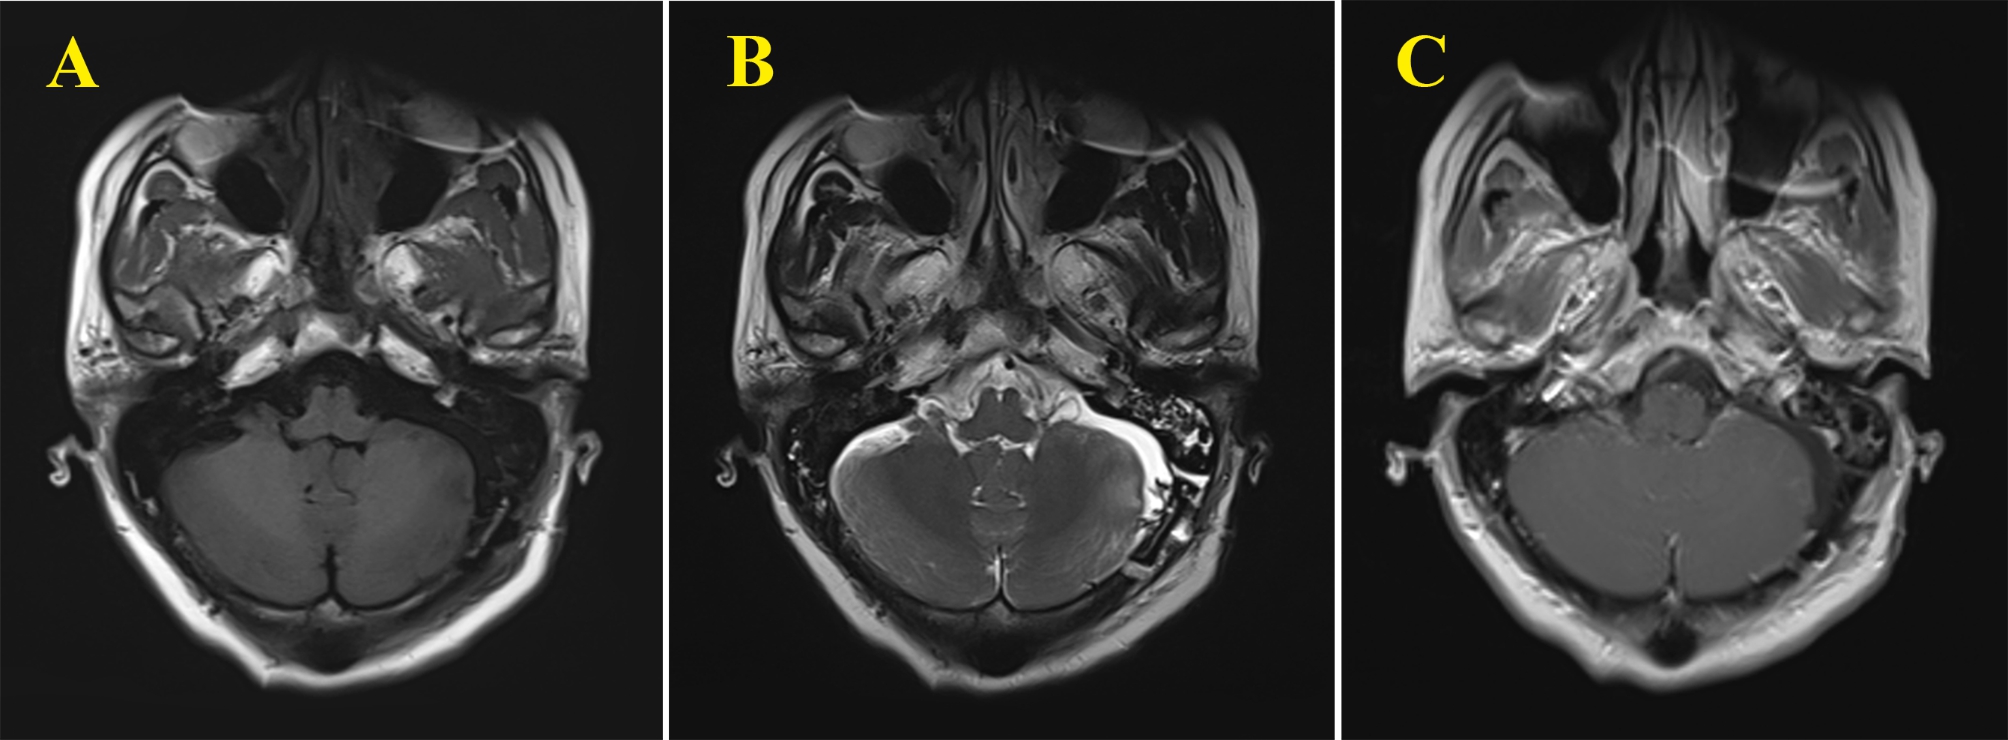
Postoperative MRI showed that the lesion had been completely removed (A, B, C)
